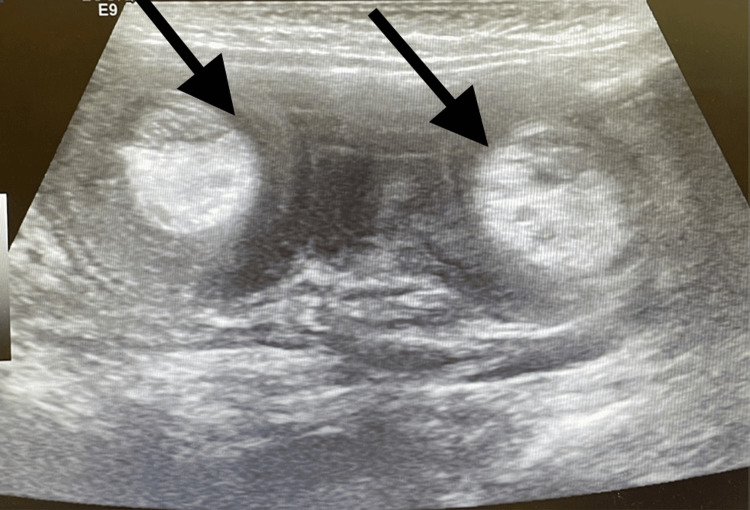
Day 1 abdominal ultrasound image showing the double target sign in the right lower quadrant
